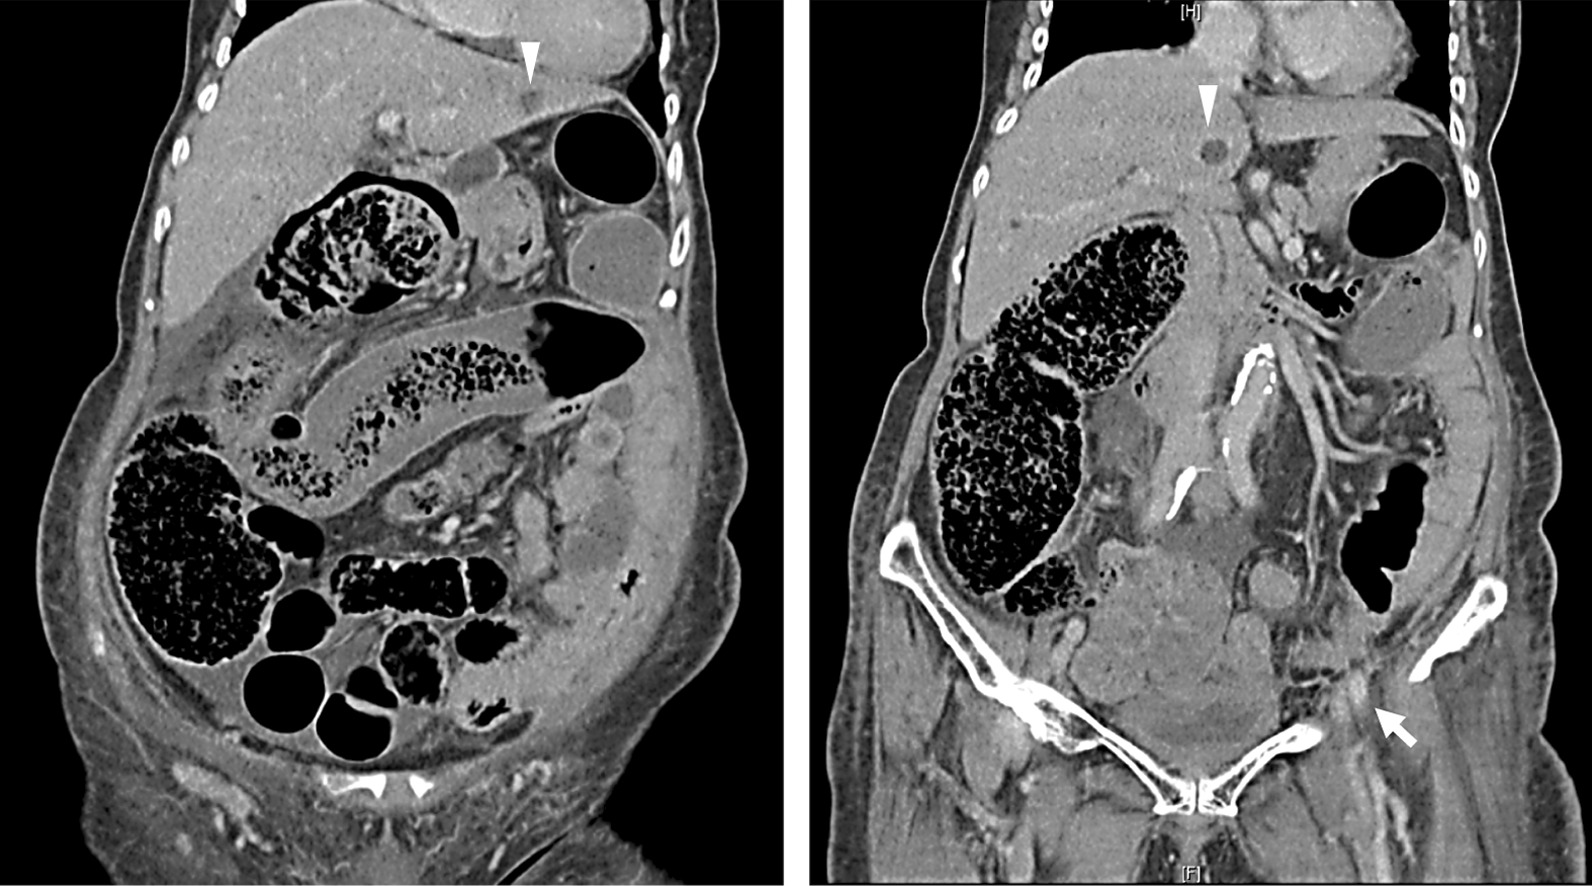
CT scan of the abdomen with/without contrast, showing distended colon with obstruction at the sigmoid colon. Increased infiltrative soft tissues near the left external iliac vessels favoring tumor metastasis (white arrow). Solid nodule in the liver (white arrow head) also suggestive of metastasis
